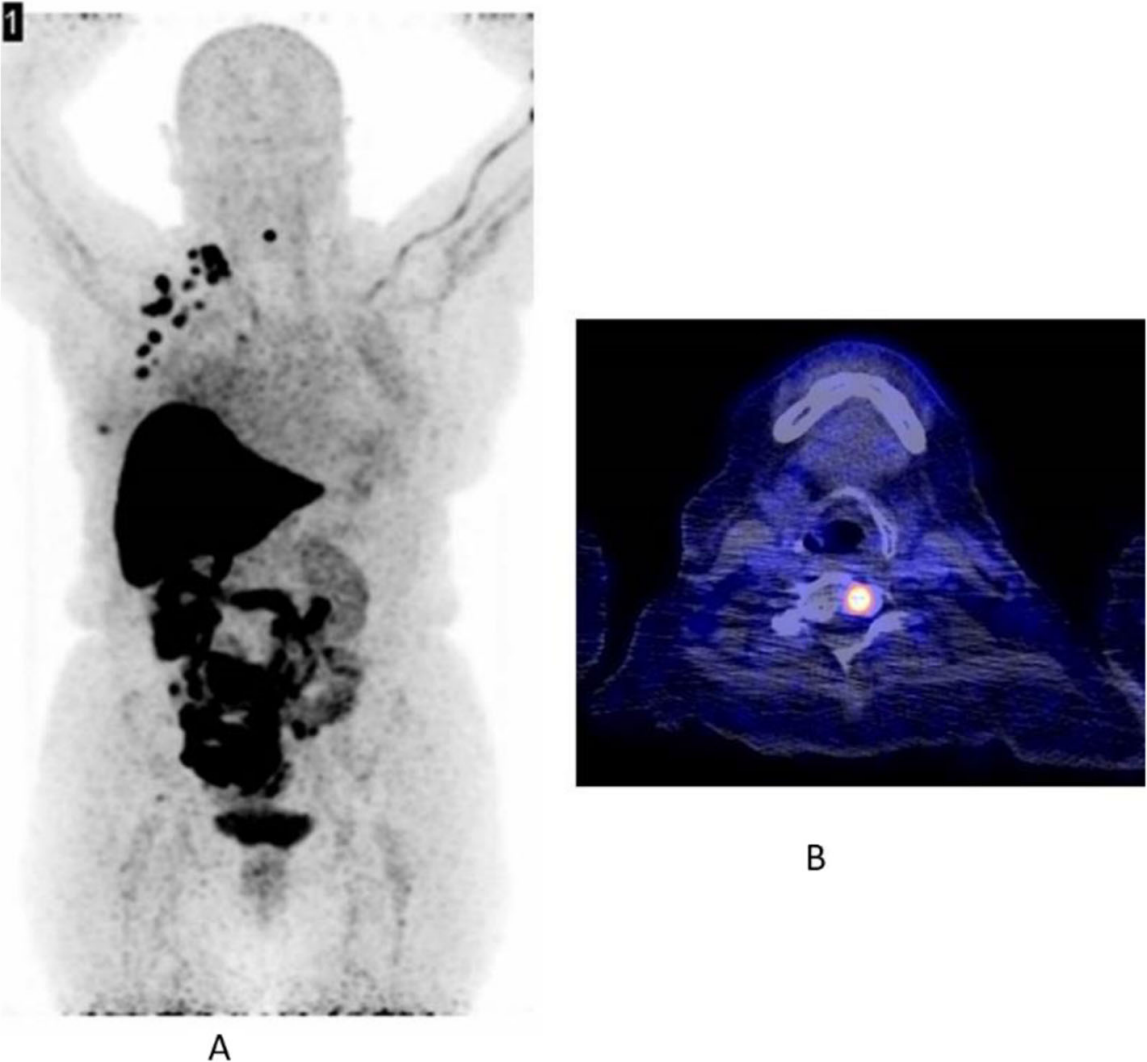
^18^F-fluoroestradiol, restaging breast cancer. *Clinical history*: 46 y.o. woman with proven breast cancer on the right side (ER+). Palpable lymph nodes in the right axilla. *PET/CT findings*: ER expression visible in the primary breast tumour and in several lymph nodes in the right axilla and in the right clavicular region in MIP (a); one focal lesion with increased ER expression in a cervical vertebra in fused images (b)
